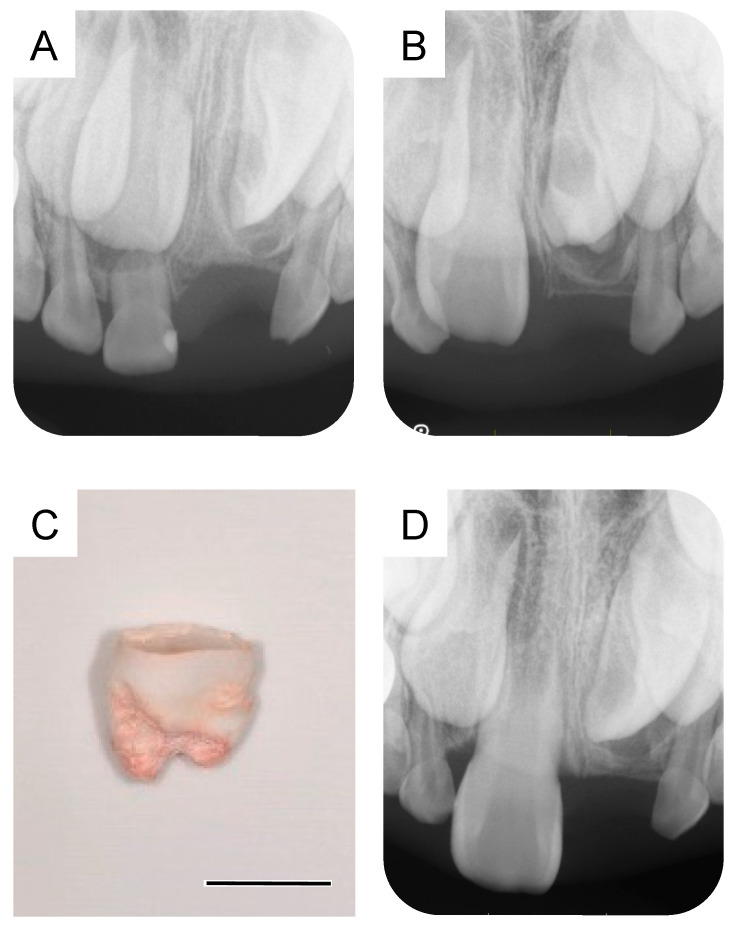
Extraction of the second supernumerary tooth. (A) Periapical radiograph after the extraction of the first supernumerary tooth at the age of 6 years and 3 months. (B) Periapical radiograph of the second supernumerary tooth detected at the age of 7 years and 5 months. (C) Extracted second supernumerary tooth at the age of 7 years and 7 months. Scale bar: 5 mm. (D) Periapical radiograph after the extraction of the second supernumerary tooth at the age of 7 years and 9 months.
